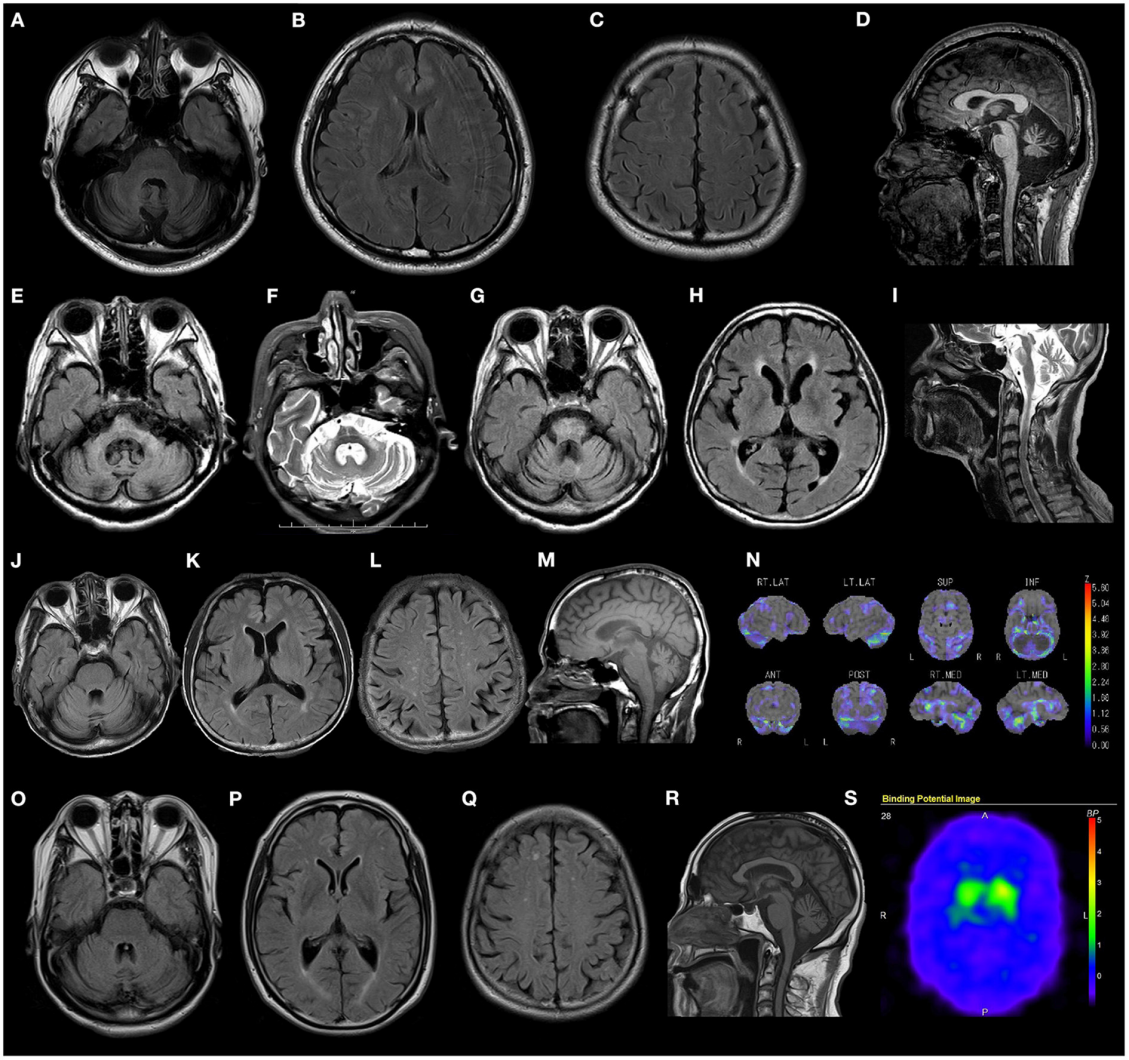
Radiological findings of patients with *RFC1* repeat expansions. (A–D) Brain MRI FLAIR images of patient 4 show marked cerebellar atrophy, without any atrophy in the brainstem and cerebrum. (E–I) Brain MRI FLAIR and T2 weighted images of patient 14 show marked cerebellar and brain stem atrophy, high T2 signal in the middle cerebellar peduncle, and hot cross bun sign. (J–N) Brain MRI FLAIR, T1 weighted images, and IMP-SPECT (decrease) images of patient 12 show slight cerebellar atrophy and decreased cerebellar blood flow, with no atrophy or hypoperfusion in the brainstem or cerebrum. (O–S) Brain MRI FLAIR images, T1 weighted images, and Datscan imaging of patient 15 show slight atrophy of the cerebellum and reduced striatal uptake of the dopamine transporter.
